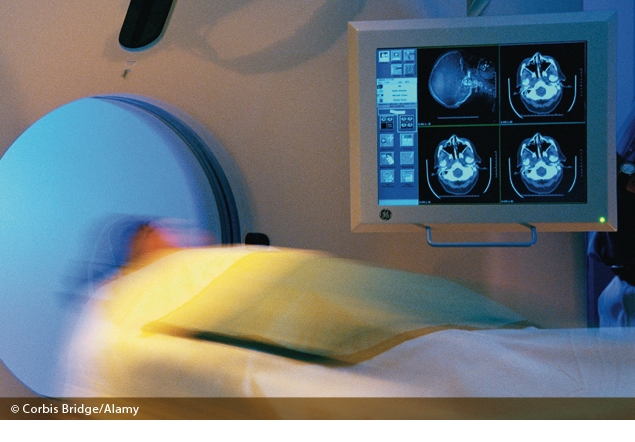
Computed tomography (CT) has been a boon for medical care. By generating detailed anatomical pictures, the technology can improve diagnoses, limit unneeded medical procedures, and enhance treatment. However, CT scans also dose patients with ionizing radiation, a known human carcinogen, posing a potential downside for public health. Mounting health worries over radiation risks are now driving efforts to limit avoidable CT scans and to reduce radiation doses where possible. “There’s a national focus on this issue right now,” says Marilyn Goske, a professor of radiology at Cincinnati Children’s Hospital Medical Center and chairwoman of the Image Gently campaign, a pediatric education and awareness campaign from the Alliance for Radiation Safety in Pediatric Imaging.
